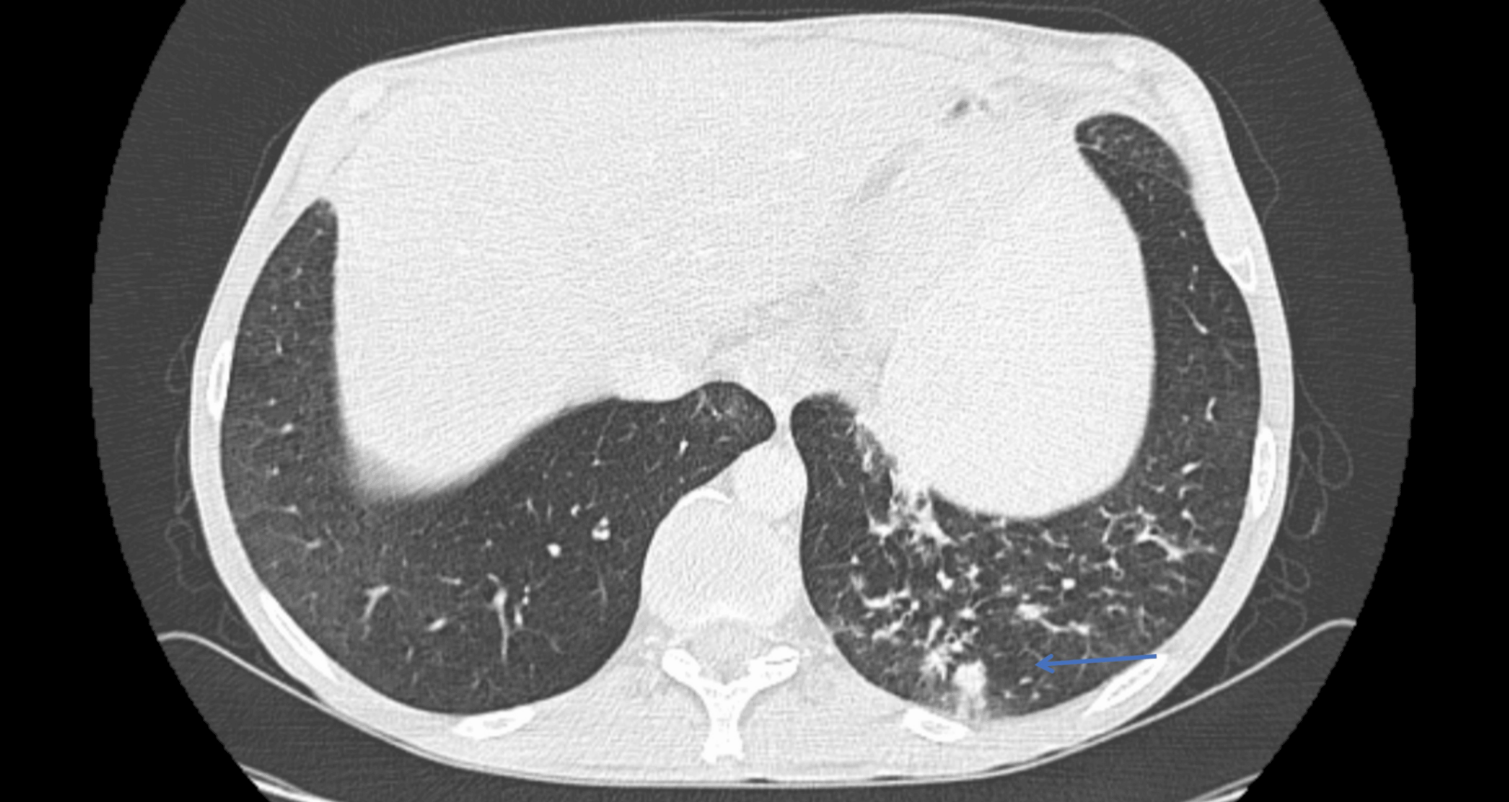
Chest CT in the axial plane showing nonspecific opacities in the left lower lobe, highlighted by the blue arrow.
